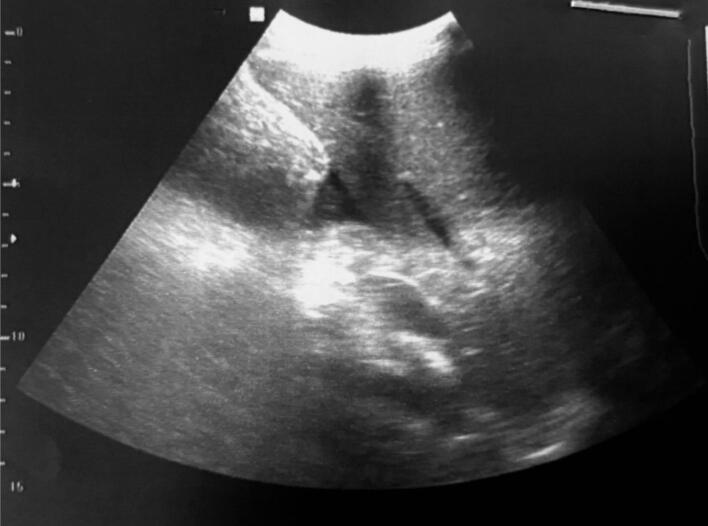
Preoperative ultrasound showed minimal free fluid.
